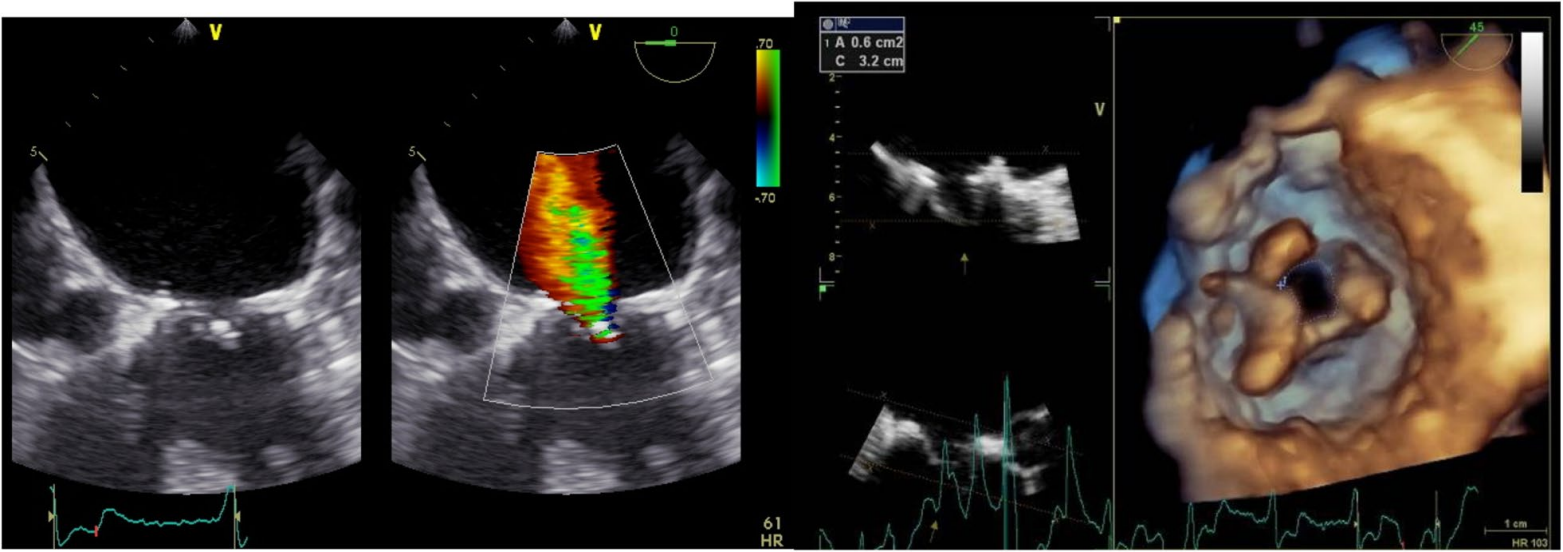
preoperative TEE showed comined severe mitral stenosis (MVA 0.6 cm^2^) and moderate valvular mitral regurge. TEE: transesophageal echokardiography, MVA: mitral valve area
